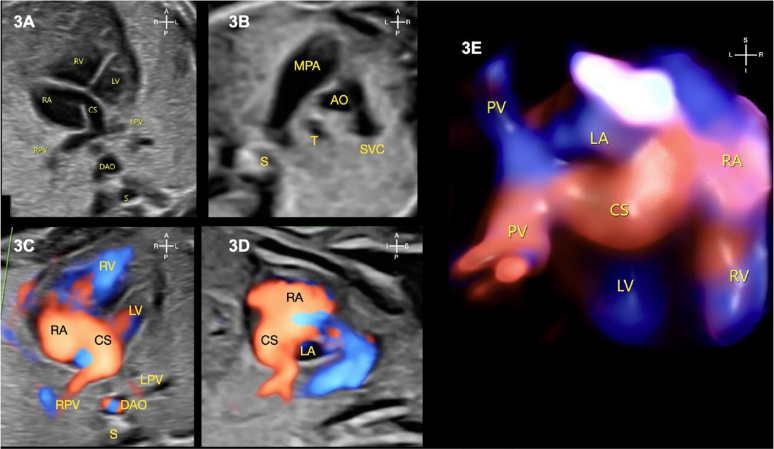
2-and 3-Dimensional Fetal Echocardiography of Coronary Sinus Total Anomalous Pulmonary Venous Connection in a 25 Weeks Fetus (A to E) CS TAPVC. (A) Four-chamber view showing dilated CS. (B) The 3-vessel view was normal. (C and D) Power Doppler 4-chamber (C) and sagittal (D) view showing the entire drainage of pulmonary veins into the CS. (E) STIC power Doppler posterior 4-chamber view showing the classic “whale tail sign” of CS TAPVC. TAPVC = total anomalous pulmonary venous connection; RPV = right pulmonary vein; other abbreviations as in Figures 1 and 2.
